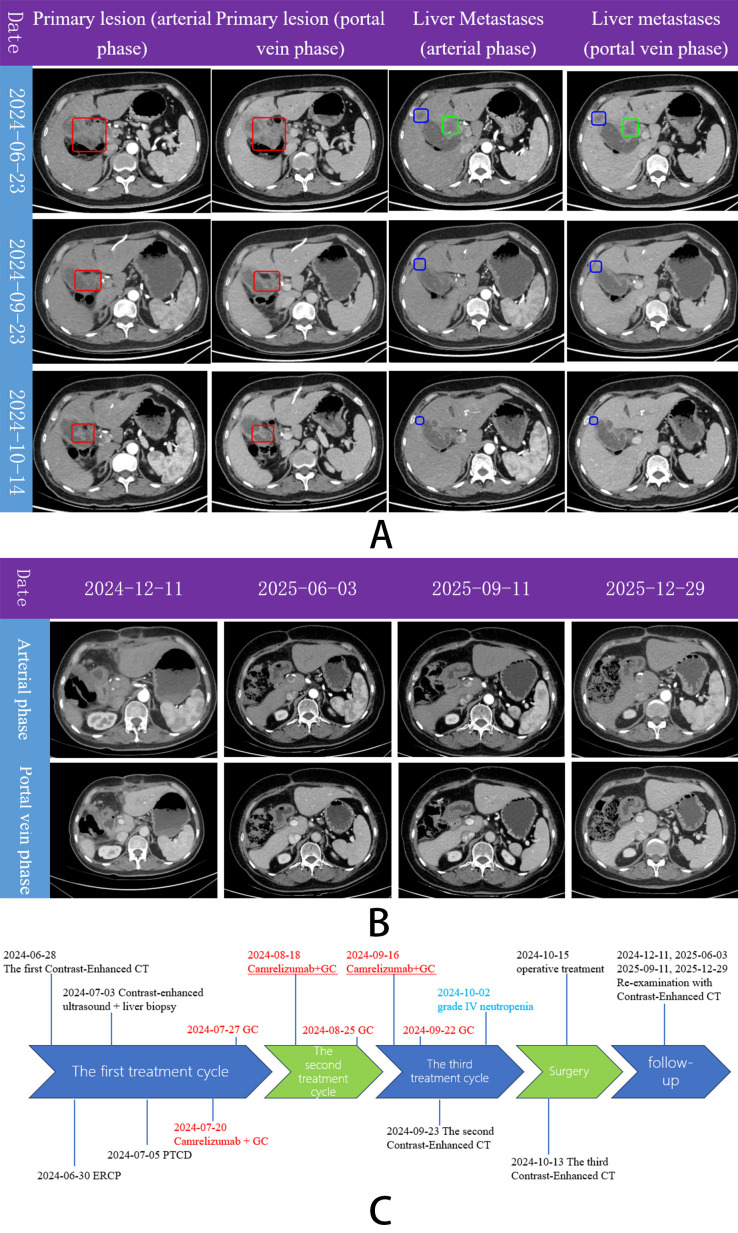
Abdominal enhanced CT scan and clinical timeline. (A) On three preoperative contrast-enhanced CT scans of the entire abdomen, the size of the primary tumor (red box) was significantly reduced in both the arterial and portal phase images, decreasing from 5.4 × 4.3 cm to 4.2 × 2.6 cm, and then to 3.3 × 2.0 cm. The size of the liver metastases (blue box) was significantly reduced in both the arterial and portal phases, decreasing from 2.0 × 1.6 cm to 1.3 × 1.0 cm, and then to 0.6 × 0.7 cm. An enlarged lymph node (green box) was visible in the hepatic hilum on the first CT scan. (B) Four postoperative follow-up enhanced abdominal CT scans demonstrated findings consistent with cholecystectomy for gallbladder carcinoma, with no abnormal enhancement and no evidence of lymph node enlargement. (C) Clinical timeline.
